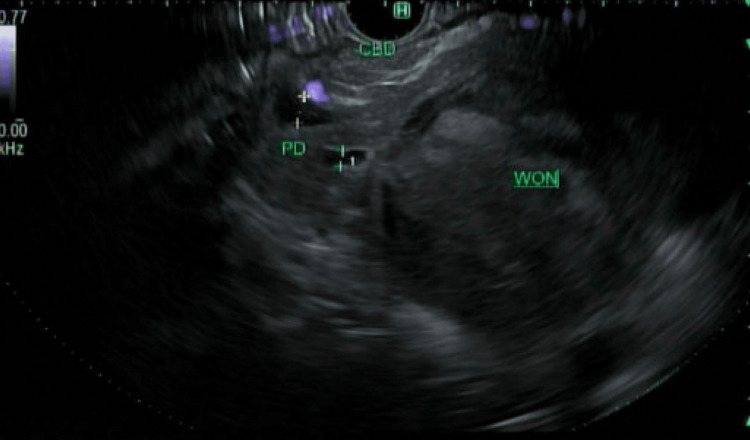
Endoscopic ultrasound image showing walled-off necrosis (WON) and active extravasation in the neck of the pancreas, suggesting pancreatic duct disconnection PD, pancreatic duct; CBD, common bile duct
